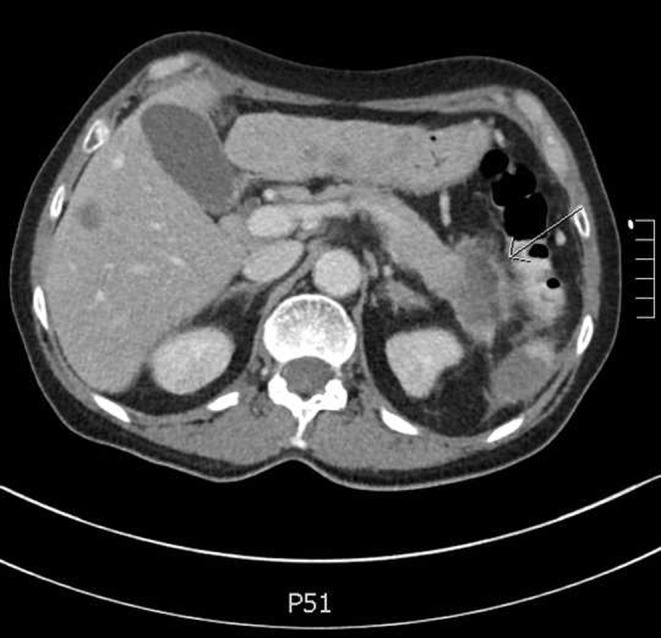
Computed tomography showing a pancreatic lesion, suspect for pancreatic cancer
